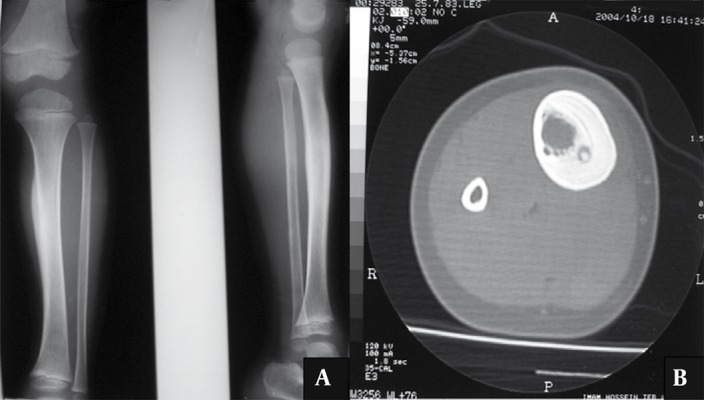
A Nine-Year-Old Boy With the Complaint of Right Shin Pain. A, The AP and lateral radiograph of the right shin revealed a solid periosteal reaction in the medial part of tibia without any obvious nidus. B, CT scan of the mid part of the right shin revealed a nidus along with a periosteal reaction around the lesion.
